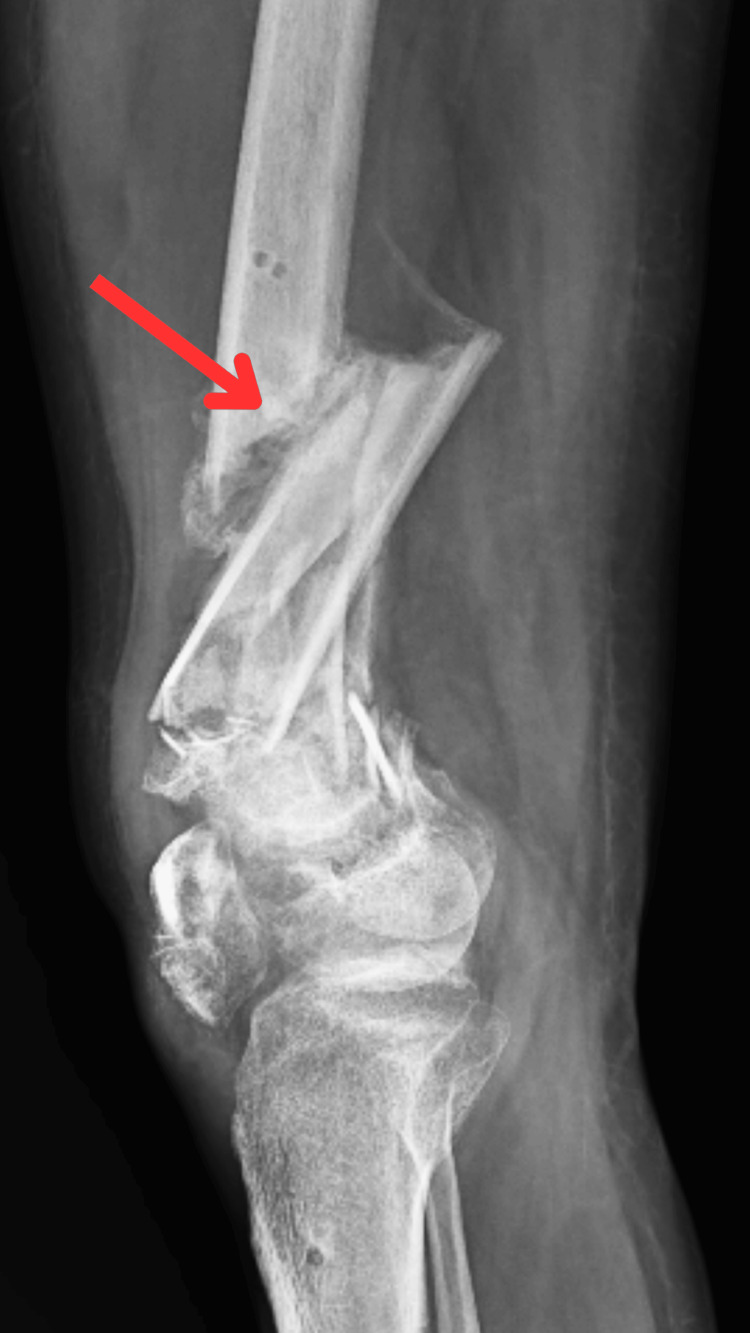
X-ray of comminuted distal femur fracture (lateral view) The red arrow points to the fracture site.
